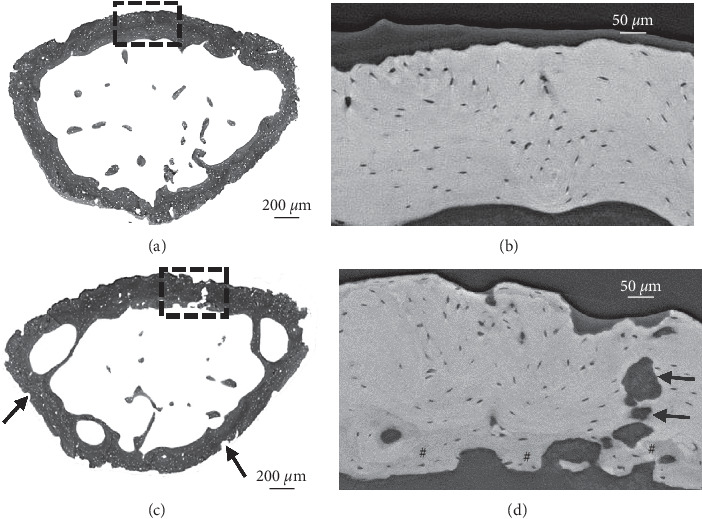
PCE-CT for bone ultrastructural characterization of femora injected with PBS (a, b) versus MOPC315.BM.Luc cells (c, d) at day 21. (a, c) PCE-CT images of cross sections of femur metaphysis. (c) Numerous sites of erosion (black arrows) and bone loss are indicated in the femur of the MM-injected bone. (b, d) High-magnification view of the rectangular area in (a) and (c). (d) Rectangular area of a local region in the cortex reveals multiple zones of intensive bone remodeling activity, with low-density new bone (darker grey, indicated with #) intermixed with mature bone sites (brighter grey), pocked with irregular-shaped “punched-out” lesions (black arrows).
